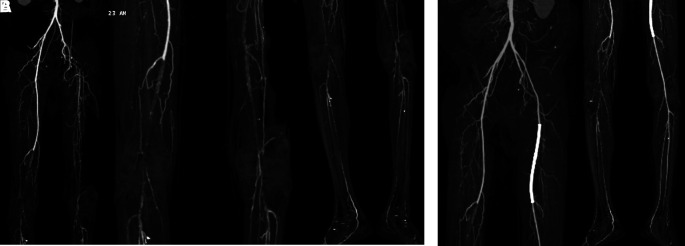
Lower extremity CT Scan Angiography of 3
